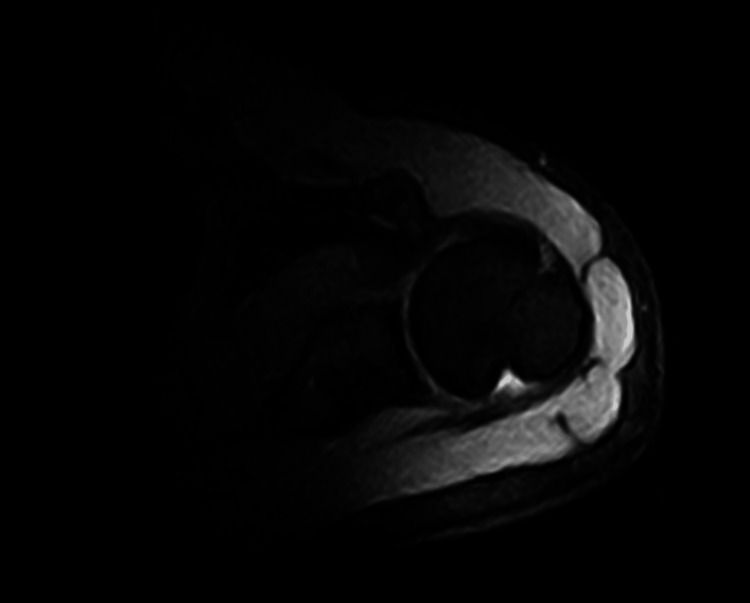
Post treatment magnetic resonance imaging (MRI) of the shoulder. The follow-up scan demonstrates evidence of healing, with reduced inflammation and signs of progressive bone remodeling.
